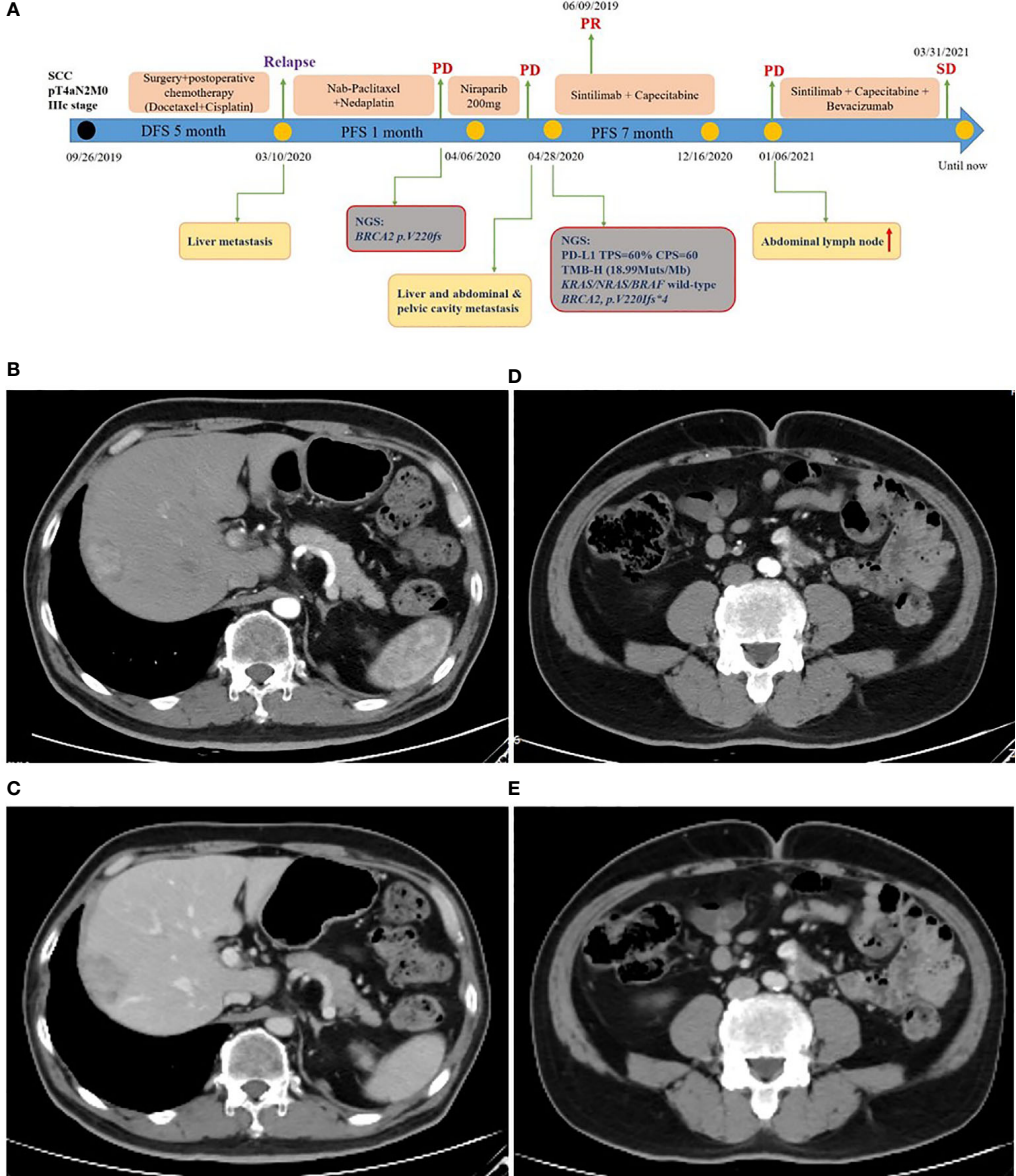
Treatment of rectosigmoid-junction SCC cancer. (A) Timeline of the clinical course in this patient. (B, C) CT scan showed a partial response in liver metastasis (40 mm × 27 mm) from arterial and portal phase images. (D, E) CT scan showed a partial response in abdominal & pelvic cavity metastasis (32 mm × 18 mm) from arterial and portal phase images.
